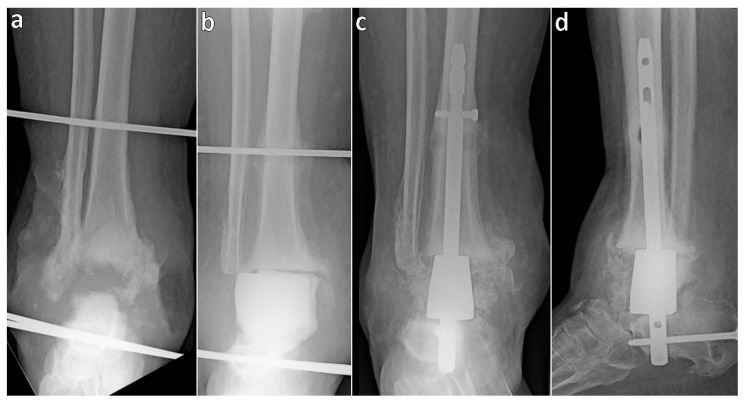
Radiographs of a patient with infected ankle nonunion: (a) after partial talus resection and external fixation at admission; (b) after complete resection and placement of a cement spacer; (c,d) final status following tibiocalcaneal arthrodesis with a trabecular metal spacer nail.
